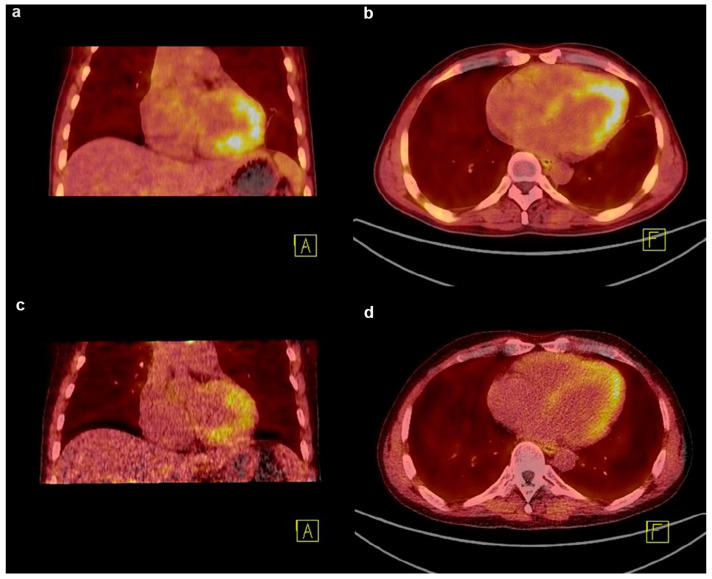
The application of [^18^F]F-FAPI PET in the response evaluation of cardiac disease. (a,b) FAPI PET/CT image of a patient with eosinophilic leukemia showing an intense uptake in the myocardium at baseline; (c,d) after 2 months of imatinib and prednisolone treatment, the follow-up FAPI PET image shows a decreased uptake in the corresponding area. Adapted from Ref. [58].
